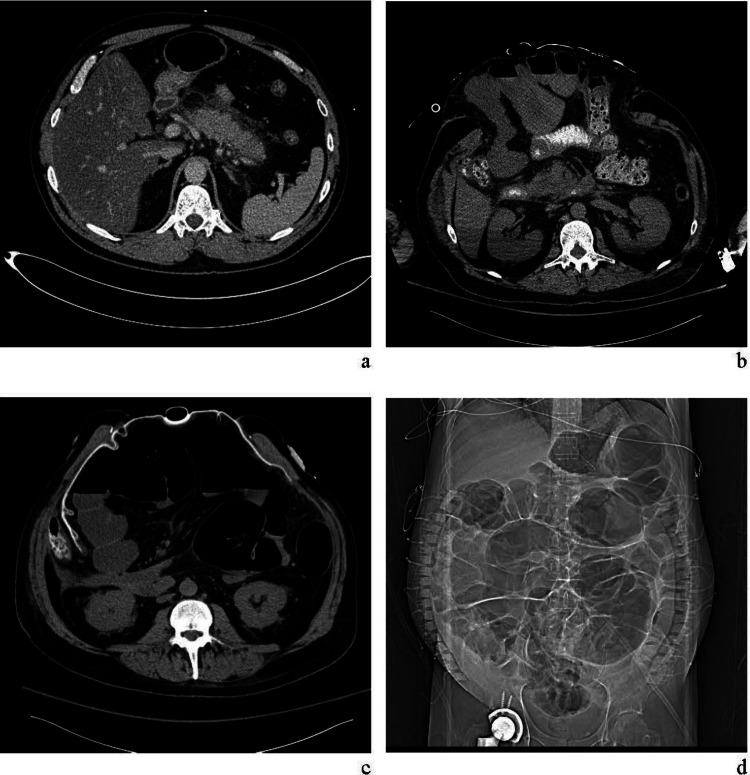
(a) Initial CT scan showing pancreatitis; (b) CT scan showing persistent edema and abdomen distension; (c) CT scan showing persistent ileus and edema and ABThera™; (d) abdominal x-ray showing ileus and ABRA® bands. CT: Computed tomography
